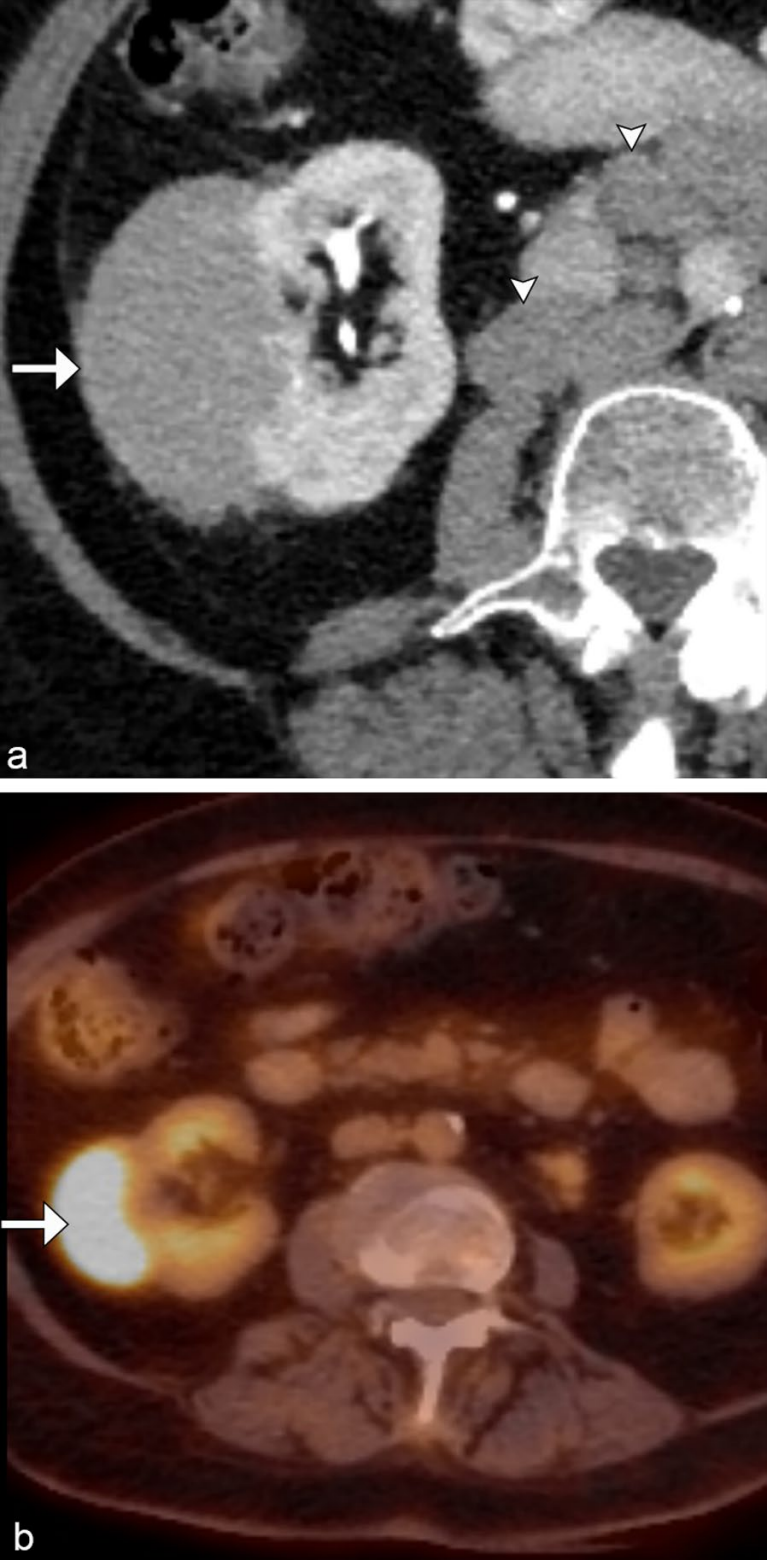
Solitary mass of renal lymphoma.* Axial contrast CT (a) demonstrates a solitary mass (arrow) in the right kidney with avid radiotracer uptake on F18-FDG PET/CT (b), as well as retroperitoneal adenopathy (arrowhead). This is consistent with solitary renal lymphomatous mass in this patient with marginal zone lymphoma. The marked FDG avidity differentiates it from typical renal cell carcinoma which can be mildly FDG-avid
